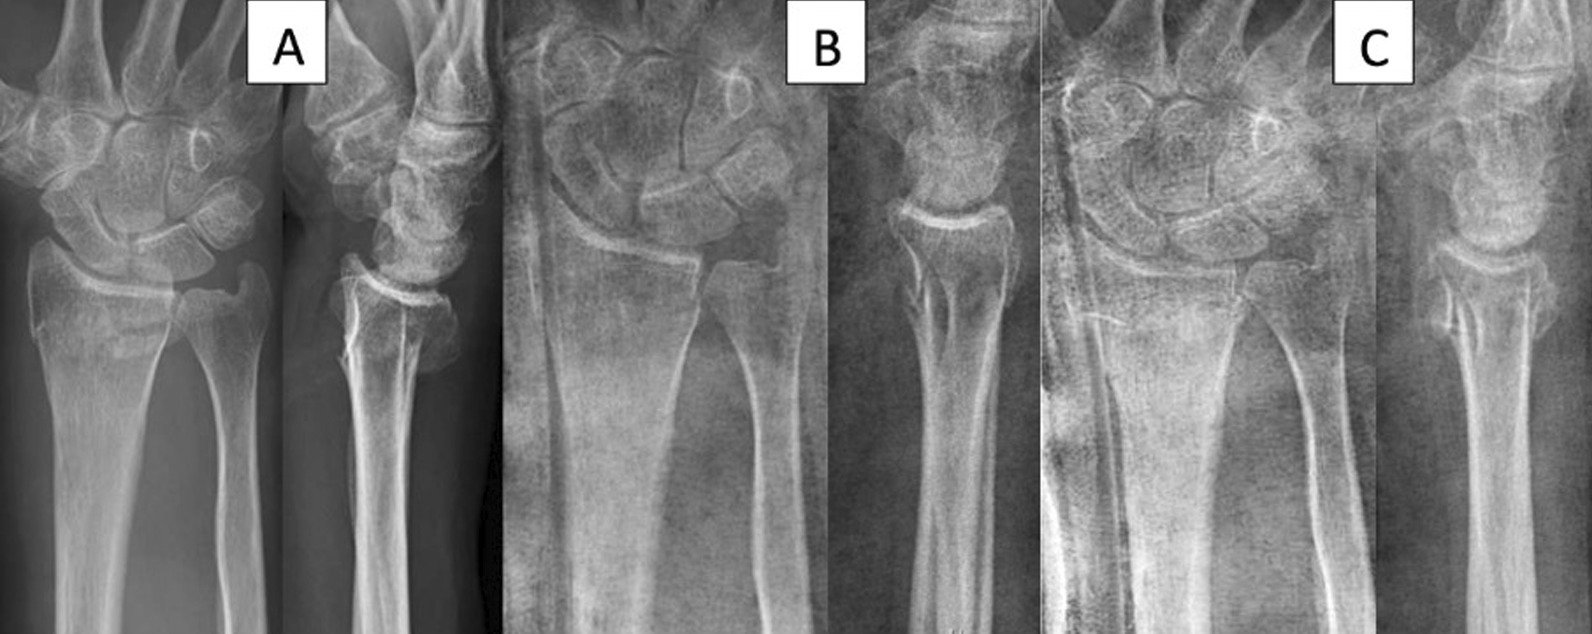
Example radiographs. 73 yo female, fall from own height on extended wrist. Pre (A) and post (B) reduction, and at follow-up (six weeks, C). Compared to pre-reduction, dorsal tilt has improved, but radius has become slightly shorter at follow-up. Reprinted with patient´s permission. Department of Radiology, Oslo University Hospital
